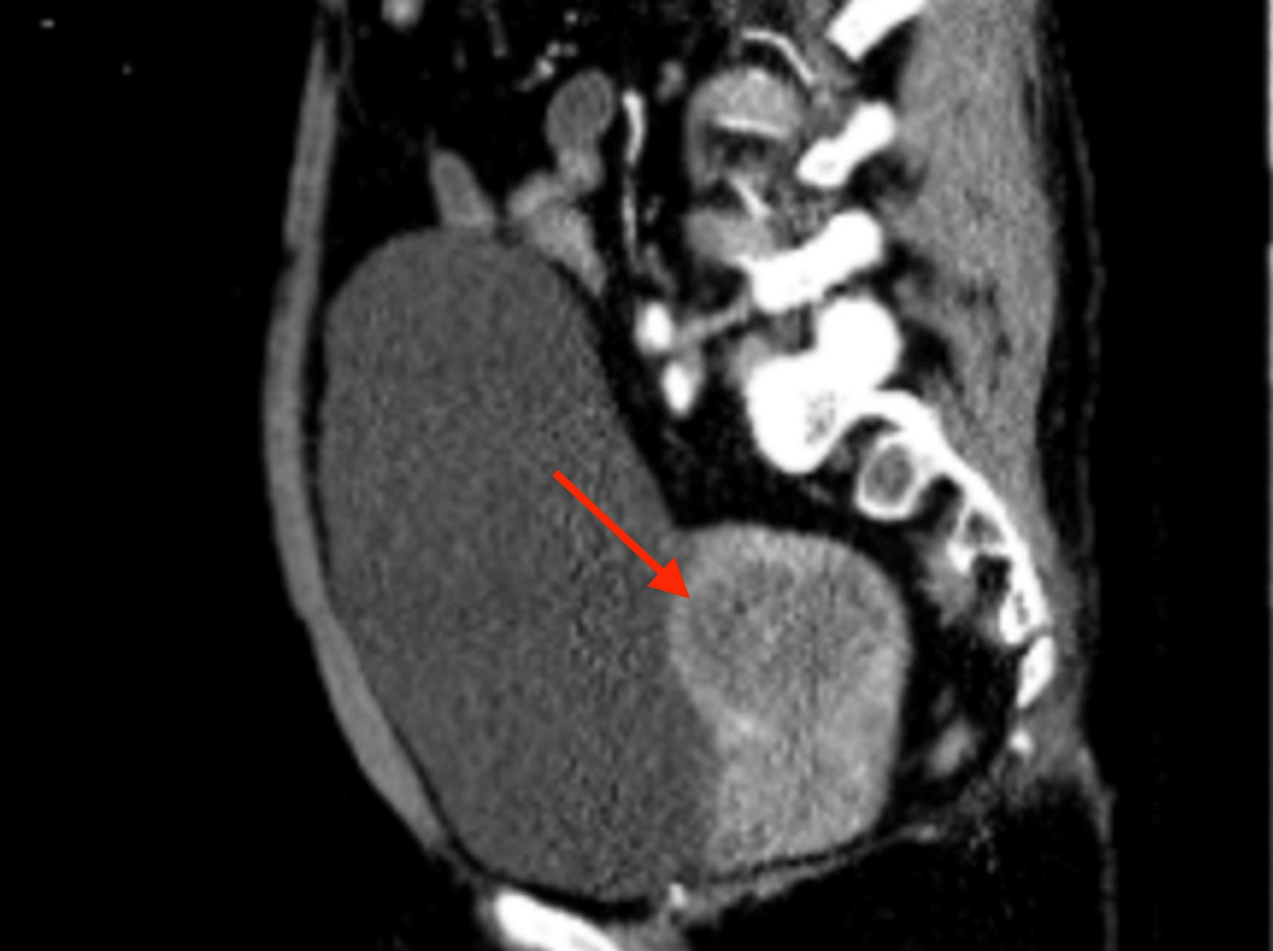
Sagittal CT abdomen and pelvis Hypoenhancing heterogeneous irregular mass within the uterus.
